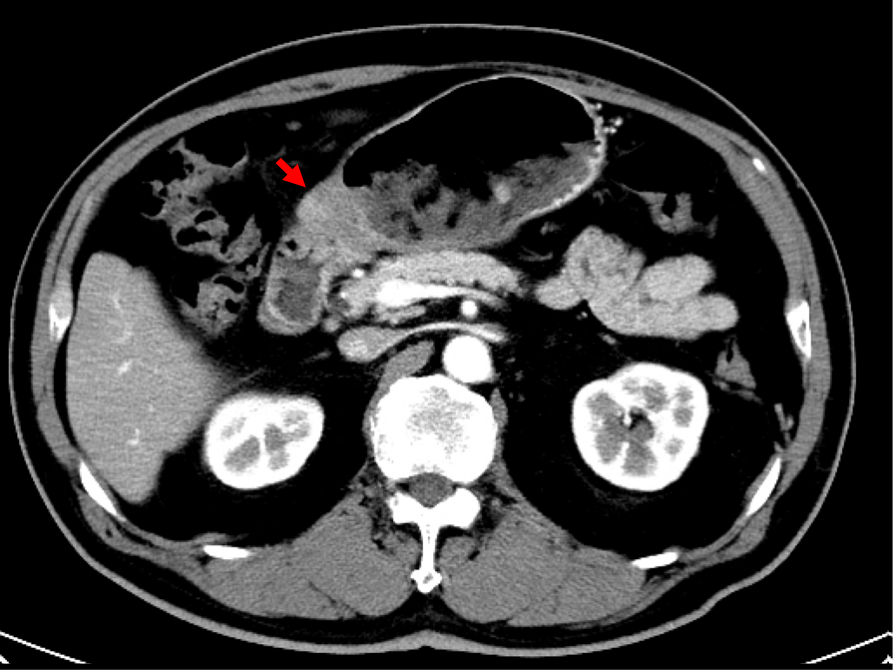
Abdominal CT scan showing a thickened wall in the first portion of the duodenum (arrow)
